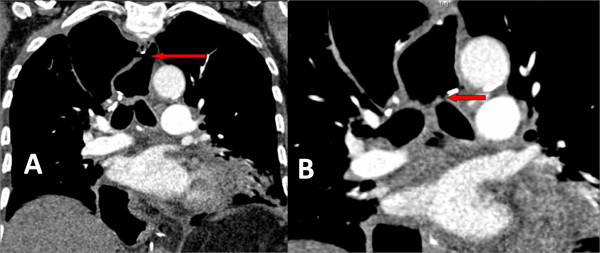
CT thorax showing the mediastinal collection of air with a single, long fistulous communication between the esophagogastric anastomosis located the neck (A) and the left main bronchus (B).
